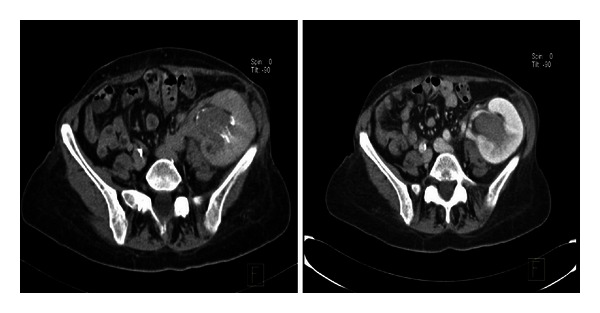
Pelvic CT demonstrating the presence of a big mass in the allograft, located in left iliac fossa, affecting renal pelvis and calyx.
